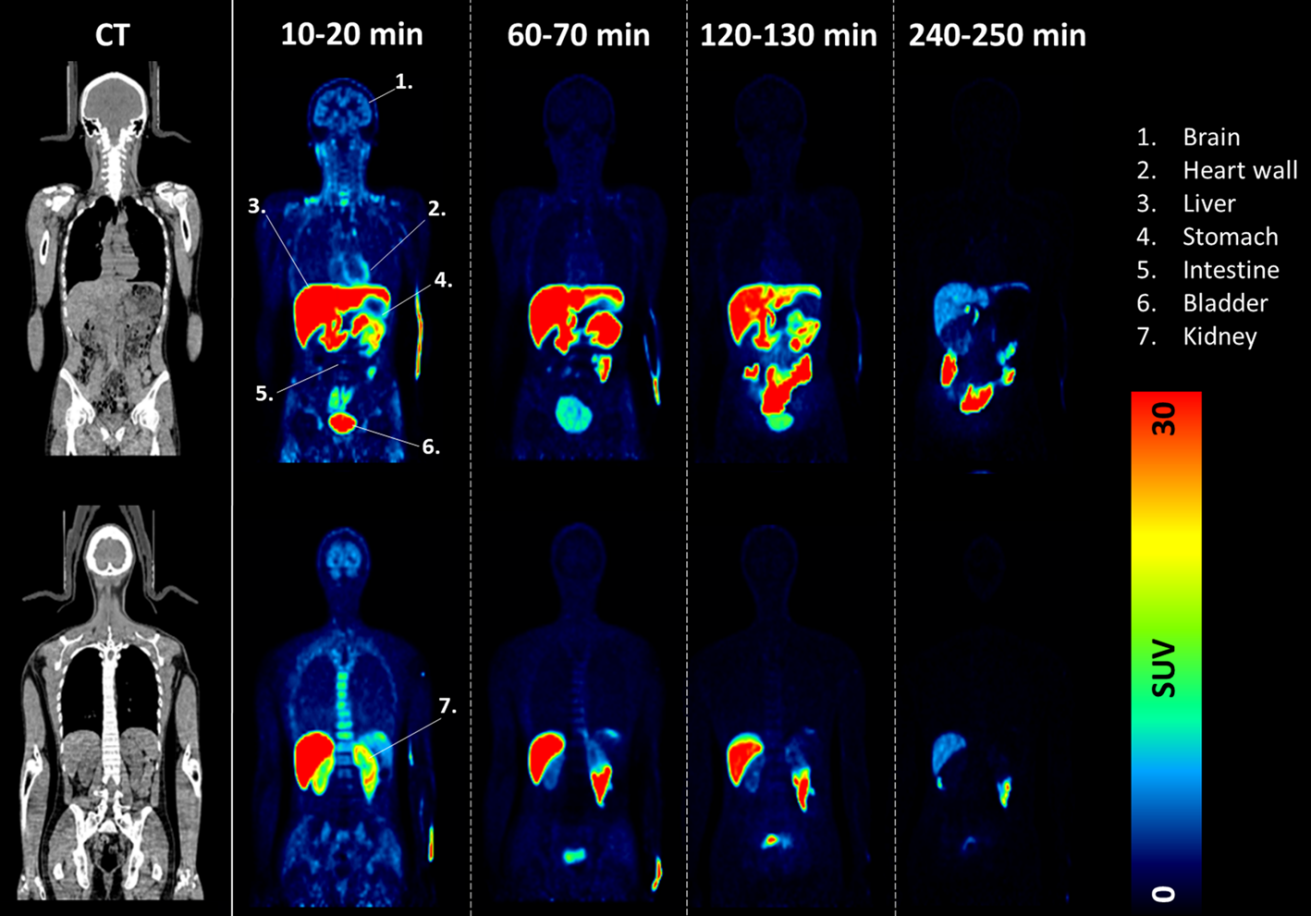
Representative dynamic whole-body PET images (15, 65, 125, and 245 min post-injection) of one healthy control in two coronal views. Images are displayed in the SUV scale
